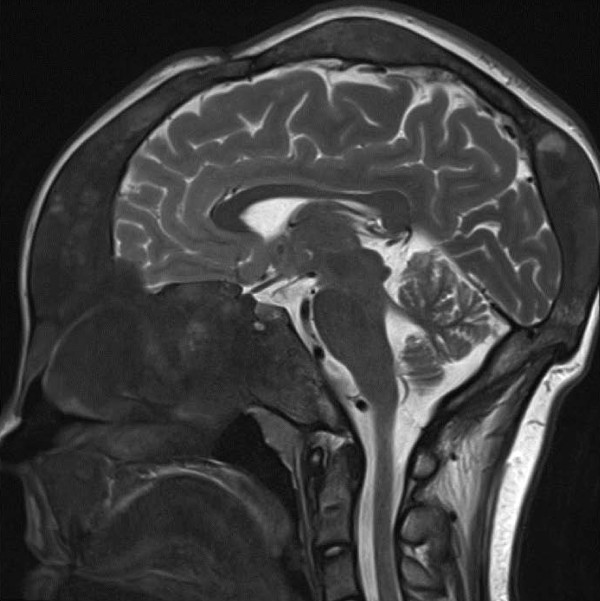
T2-weighed sagittal magnetic resonance image. Shows massive thickening of her skull base due to fibrous dysplasia and a pituitary adenoma.
